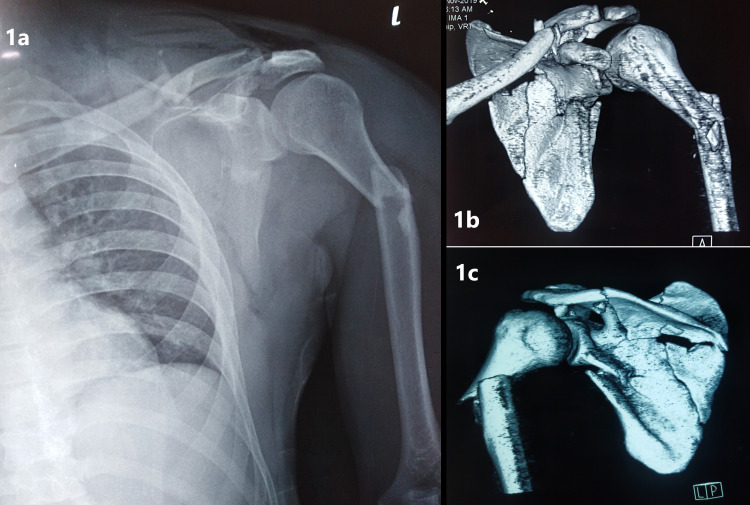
Anteroposterior (AP) radiograph and 3D-reconstructed CT scan images of the left shoulder. (a) The preoperative radiograph showing the anteroposterior (AP) view of the left shoulder clearly enabled the establishment of the diagnosis of the ipsilateral lateral third clavicle, comminuted scapula and proximal third humerus fractures. (b,c) Detailed assessment using CT scan showed both the anterior and posterior surfaces of the left shoulder in the coronal plane. The extension of the fractures can be clearly seen, especially for the scapula, which outlined the highly comminuted scapular fractures involving the scapular body, segmental fracture of its medial border, infraglenoid fracture of its lateral border and fracture of the scapular spine at the base of the acromion. This is indispensable for preoperative planning and preparation.
